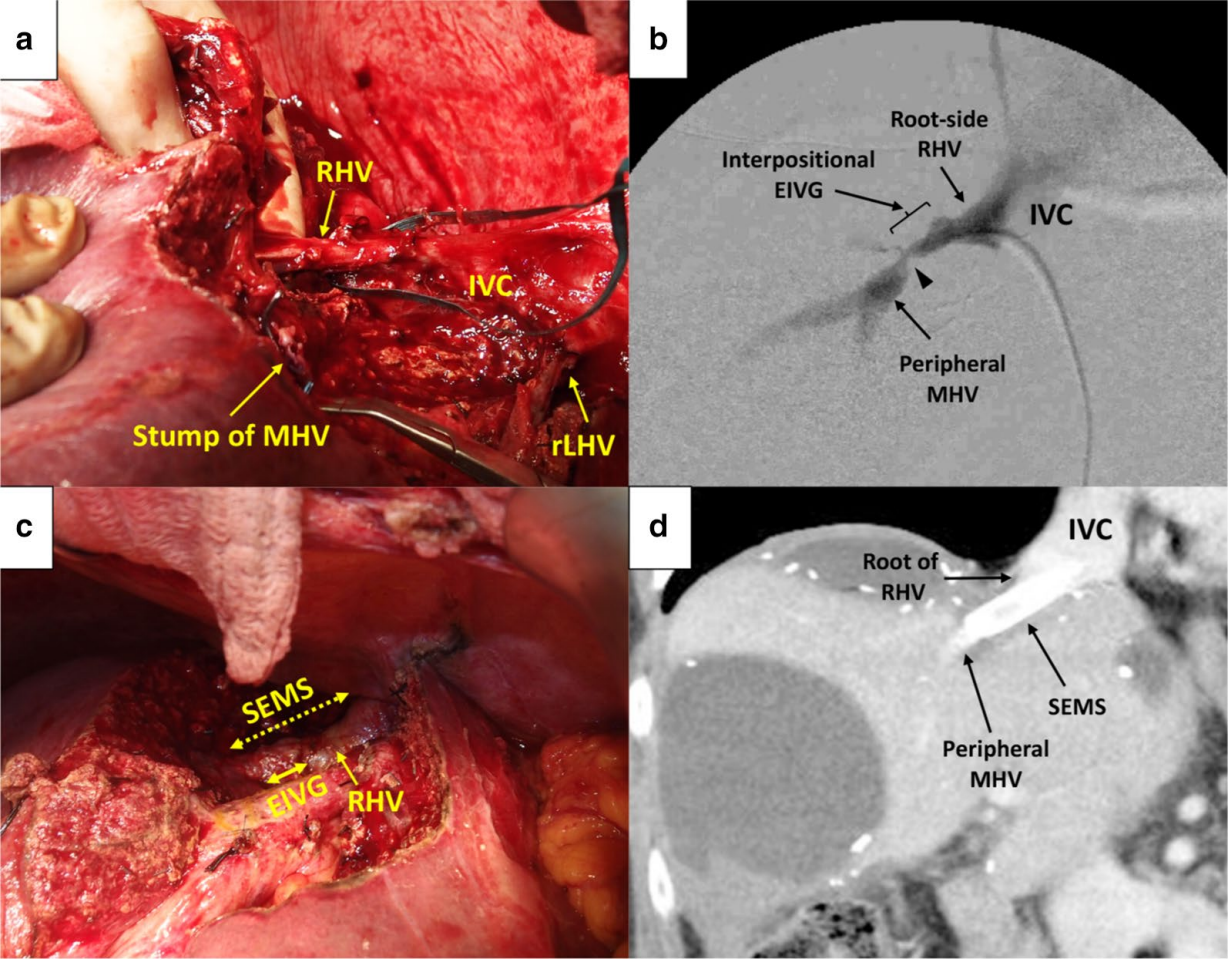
Intraoperative findings (a–c) and a postoperative computed tomography (CT) image (d) (Case 1). a A picture showing the operative field after a segment S4/8 non-anatomic hepatectomy with combined resection of the root-side part of the MHV. Note the clamped stump of the MHV. Inferior vena cava (IVC) and the RHV were exposed. The LHV was already partially resected and reconstructed (rLHV) after an anatomic segment 3 resection for another tumor. b Intraoperative hepatic venogram showing a severe stricture at the anastomosis between the interpositional EIVG and peripheral MHV (arrowhead). c A picture showing the operative field after deployment of SEMS (dotted two-way arrow) inside the HV reconstruction from the RHV to the peripheral MHV across the anastomosis using an EIVG (solid two-way arrow). d A CT image at 8 months postoperatively showing a fully patent SEMS with sufficient MHV outflow
